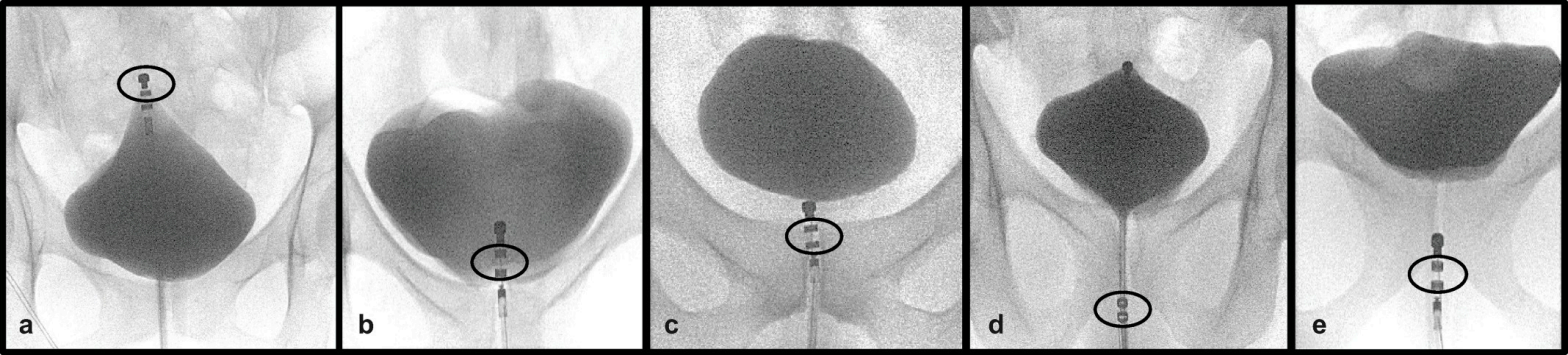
Fluoroscopic images for catheter positioning at different stimulation locations. Examples of fluoroscopic images taken after catheter positioning at the five specific stimulation locations: Bladder dome (BD, 2a), trigone (TG, 2b), proximal urethra (pUR, 2c), membranous urethra (mUR, 2d), distal urethra (dUR, 2e). Bladder volume was 60mL of contrast medium. Radiographs 2a, 2b, and 2e show positioning of the catheter for stimulation in female lower urinary tract (LUT), while 2c and 2d represent images of the catheter in male LUT. The stimulating electrodes are encircled in black.
